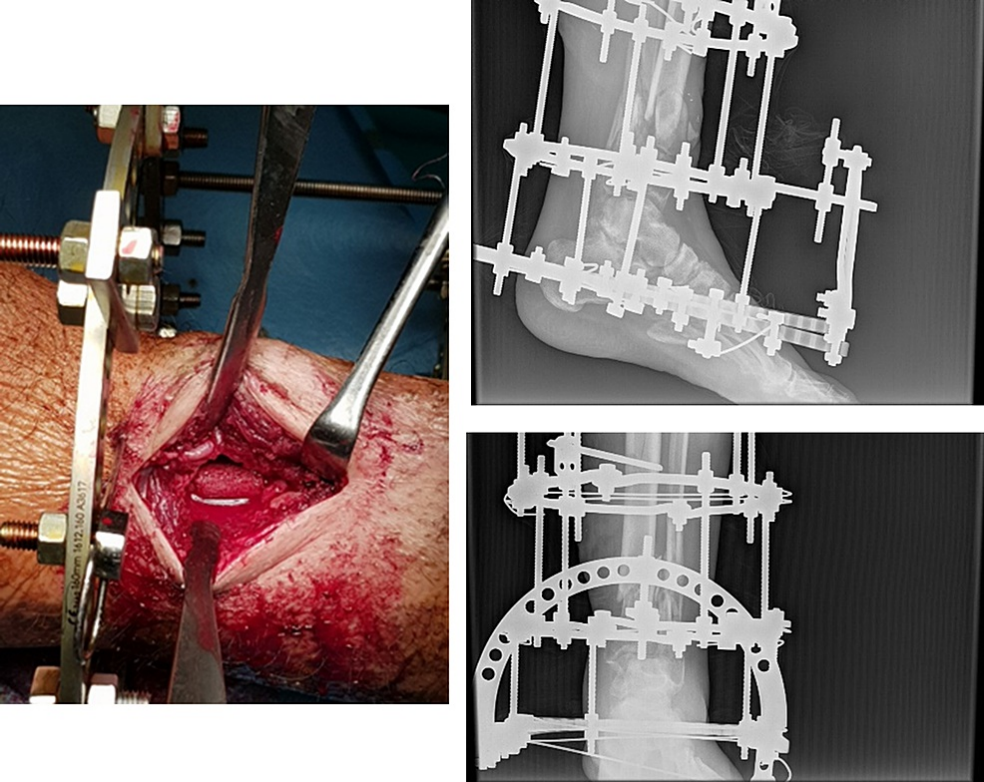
Iliac autografts cylinders placement and post-operative x-rays.
